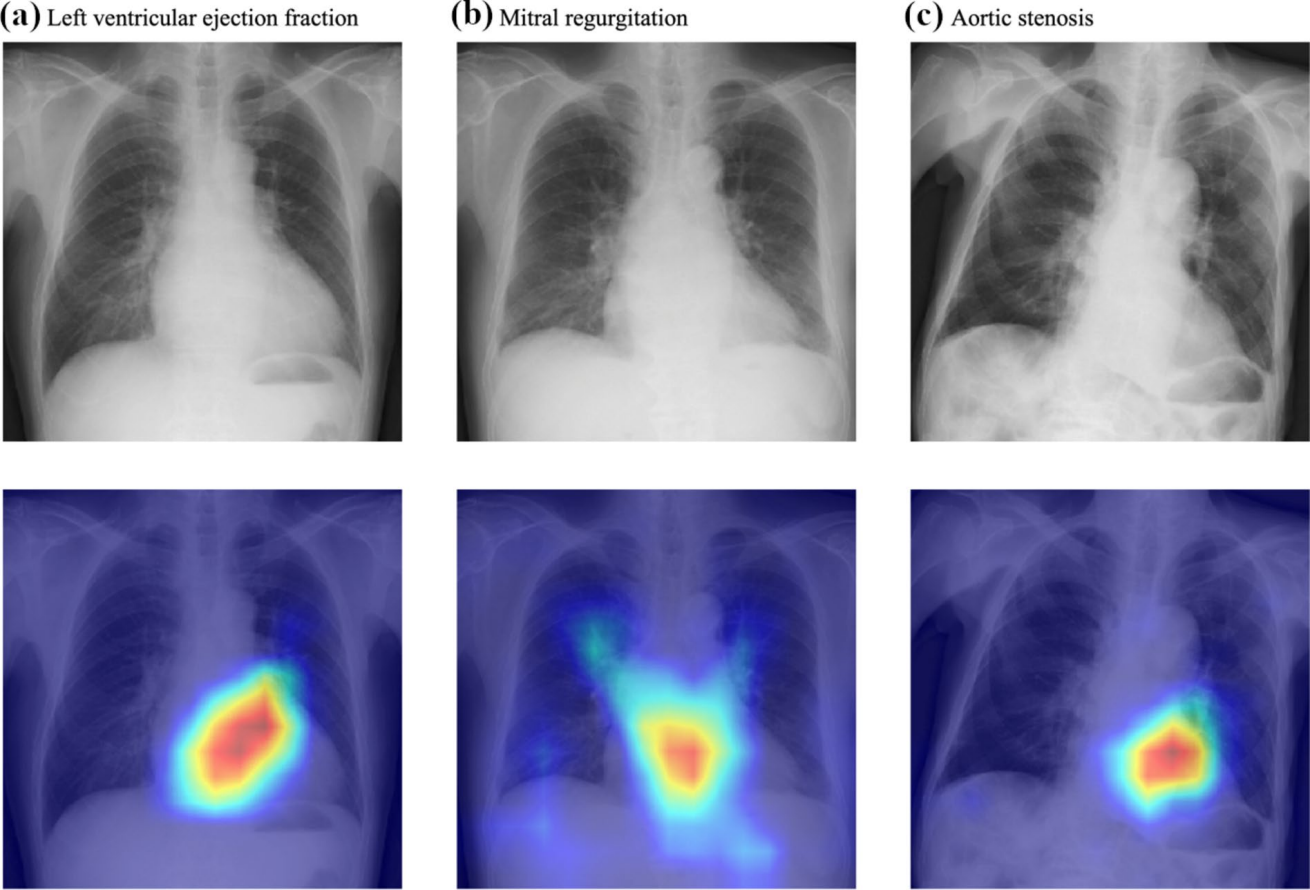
Chest radiographs (above) and corresponding saliency maps (below) demonstrating three different cardiac conditions. a Low left ventricular ejection fraction: the chest radiograph shows cardiomegaly. The saliency map highlights the cardiac short axis with high intensity (red–yellow). b Mitral regurgitation: The chest radiograph demonstrates an enlarged cardiac silhouette with prominent pulmonary vasculature. The saliency map shows intense activation from the central cardiac region to the hilar region. c Aortic stenosis: the chest radiograph shows cardiac enlargement with possible aortic valve calcification. The saliency map’s activation is focused in the aortic region, consistent with the pathology location
